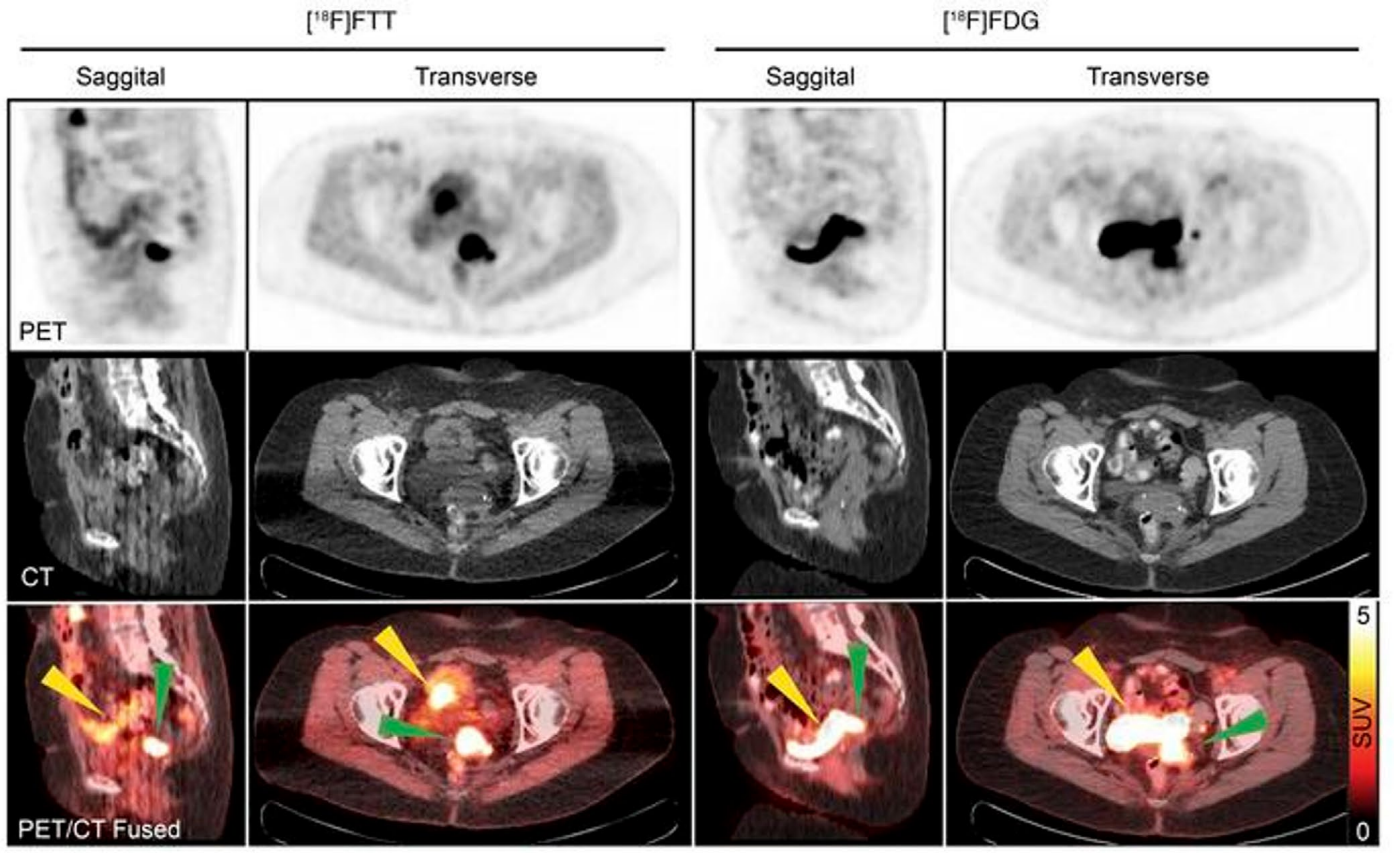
[^18^F]PARP1‐inhibitor and [^18^F]FDG PET/CT images of a patient with ovarian cancer with vaginal cuff lesion. From Makvandi et al. [65] [^18^F]FTT (left) is a [^18^F]PARP1‐inhibitor. Minimal radiotracer in the urinary bladder with [^18^F]PARP1‐inhibitor PET allowed for clear visualisation of the lesion (green arrow) with no interference, despite some bowel uptake (yellow arrow on [^18^F]PARP1‐inhibitor image). Note excreted radiotracer in the bladder on [^18^F]FDG PET (yellow arrow on [^18^F]FDG PET). Copyright 2018, American Society for Clinical Investigation.
